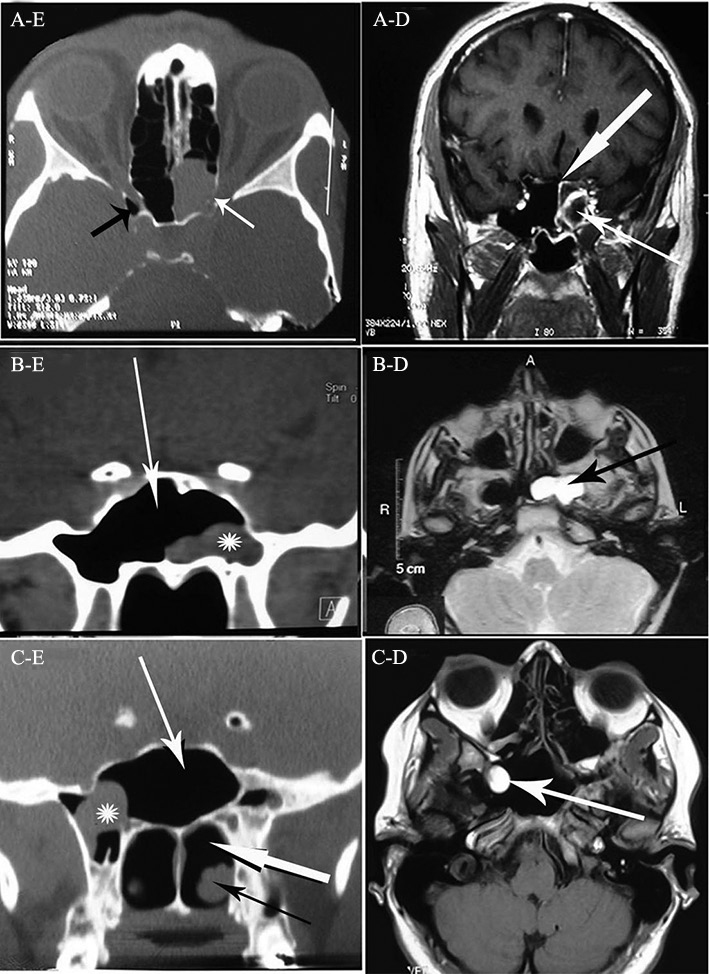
A: (Left) axial CT scan of sinuses shows complete opa-cification of the left sphenoid sinus with dehiscence in the left optic canal (white arrow), opticocarotid recess (black arrow); (Right) MRI shows the cyst with rim enhancement obstructing the sinus ostium (thin arrow), thick arrow shows enhanced sphenoid sinus mucosa. B: (Left) coronal CT scan of sinuses shows a retention cyst (astrix) in the lateral recess of sphenoid sinus, white arrow shows sphenoid sinus; (Right) T2-weighted MRI shows left sphenoid sinus hyper-intense retention cyst (black arrow). C: (Left) coronal CT scan shows sphenoid sinus (white thin arrow) and retention cyst (astrix) without pressured on the optic chiasm, posterior chornea (thick white arrow) and inferior turbinate (black arrow); (Right) MRI shows hyper-intense mass in the sphenoid sinus and its lateral recess (white arrow).
